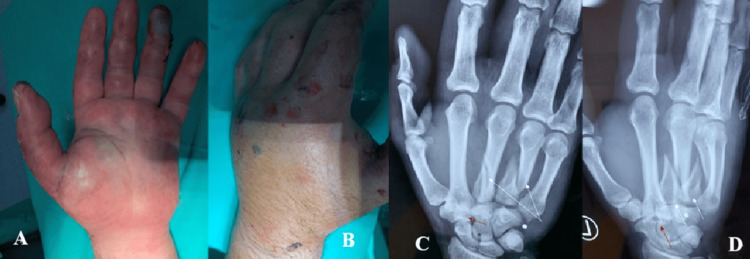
(A) Palmar and (B) dorsal surface depict the great swelling of the hand. Preoperative (C) anteroposterior and (D) oblique X-ray views show fractures of the third and fourth metacarpals (white arrows) and a fracture-dislocation of the base of the second metacarpal (red arrow).
